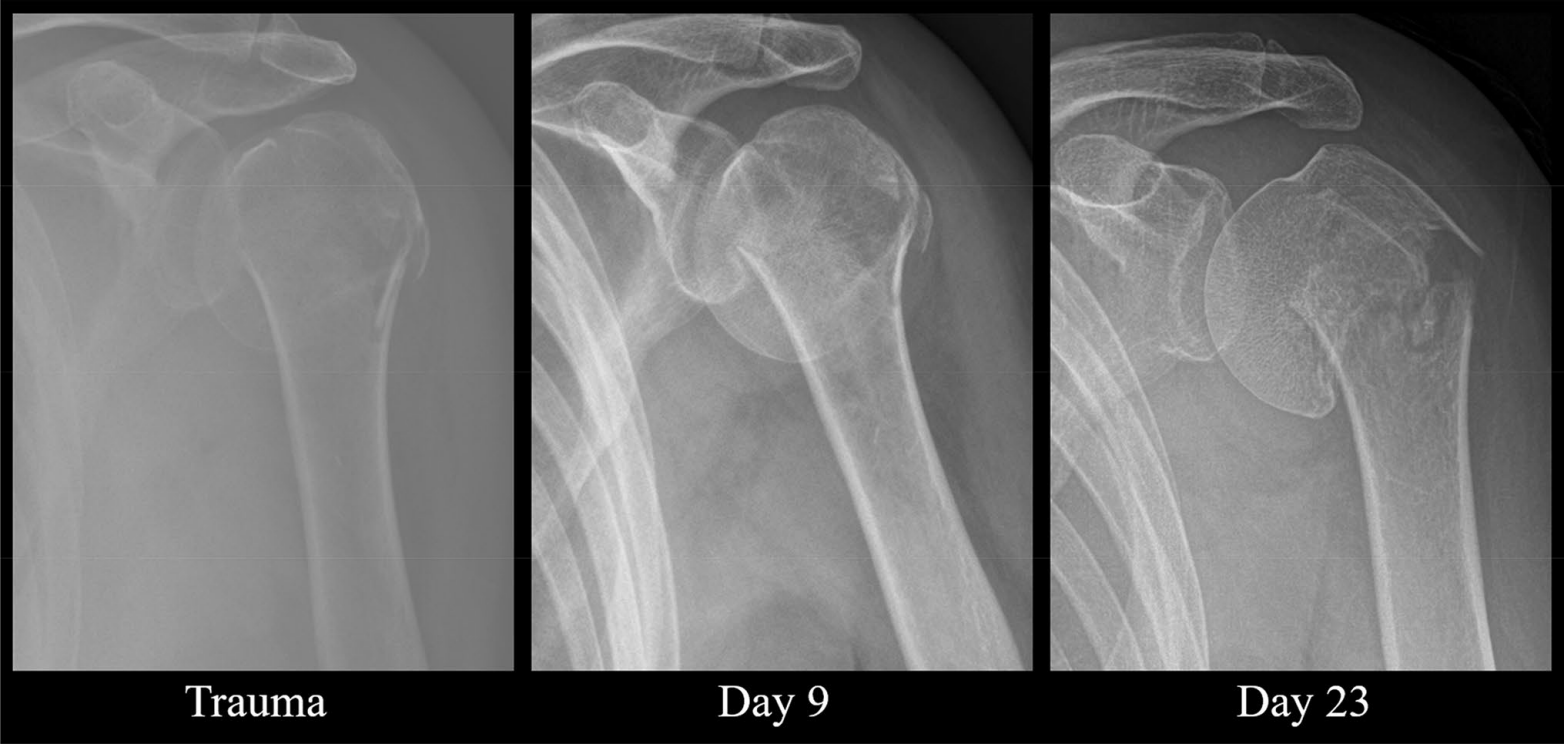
Radiographic follow-up of a type C fracture. Trauma: NSA = 103.8°, MMG = 28.1 mm, MLG = 2.1 mm. Day 9: NSA = 98.1°, MMG = 36.7 mm, MLG = 0.0 mm. Day 23: NSA = 88.0°, MMG = 18.1 mm, MLG = 14.3 mm
